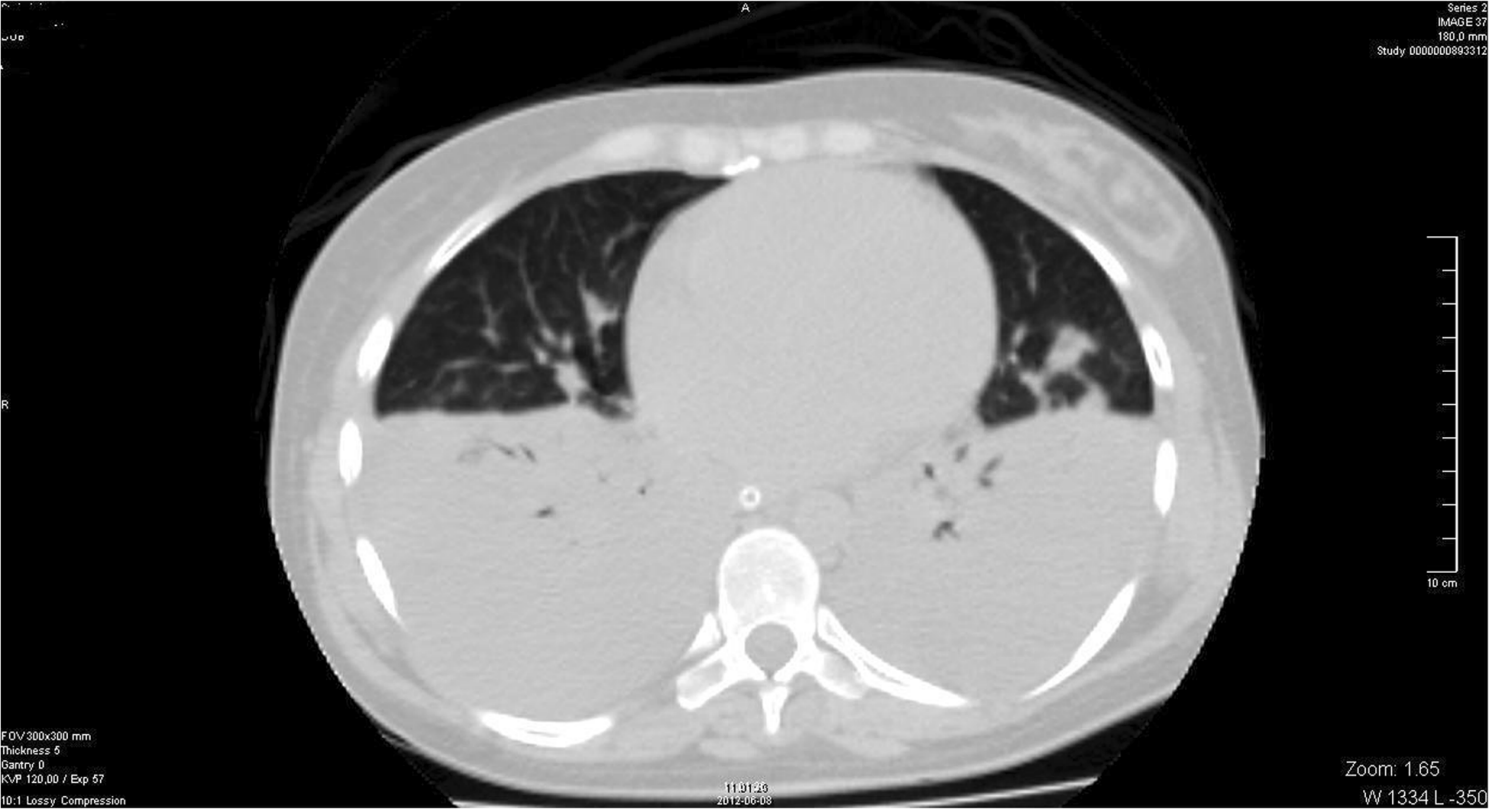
Chest computed tomography demonstrating severe pulmonary oedema
